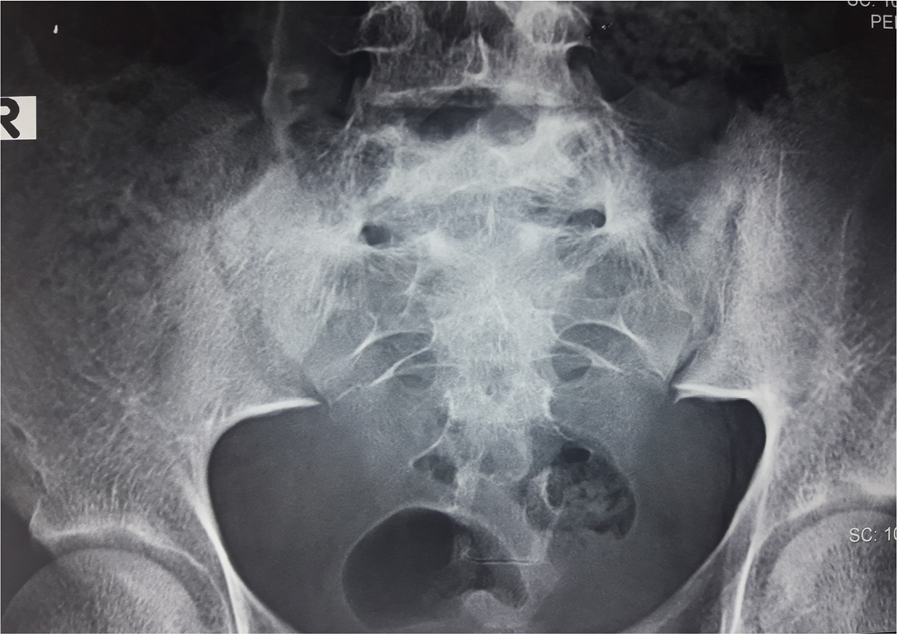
X-ray of the sacroiliac joint showing features of right-sided sacroiliitis with joint space widening and reactive bony changes surrounding the joint
